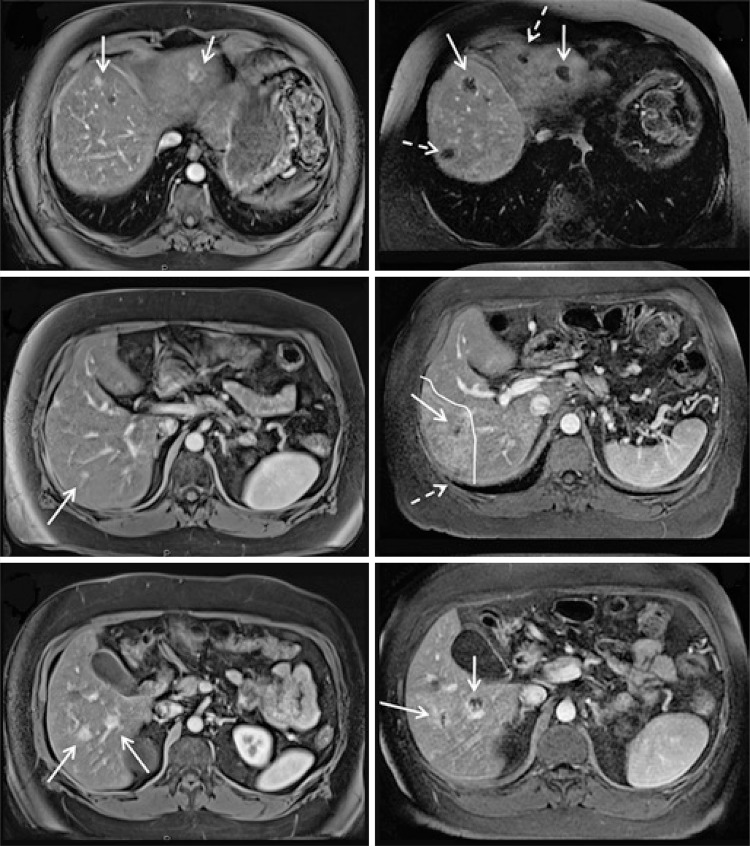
Upper abdomen magnetic resonance, T1-weighted with fat saturation after contrast, in portal phase, axial section, showing secondary hepatic lesions in (A) segments II and IVa (white arrows), (B) segment VII (white arrow) and (C) segment VI (white arrows). Upper abdomen magnetic resonance, T1-weighted with fat saturation after contrast, in portal phase, axial section, showing secondary hepatic lesions in (D) segments II and IVa (white arrows), (E) segment VII (white arrow) and (F) segment VI (white arrows). There are necrotic areas, which showed no lesions in a previous study: images D and E (dotted white arrows) and image E, area of hyperenhancement in segment VII (white arrow)
